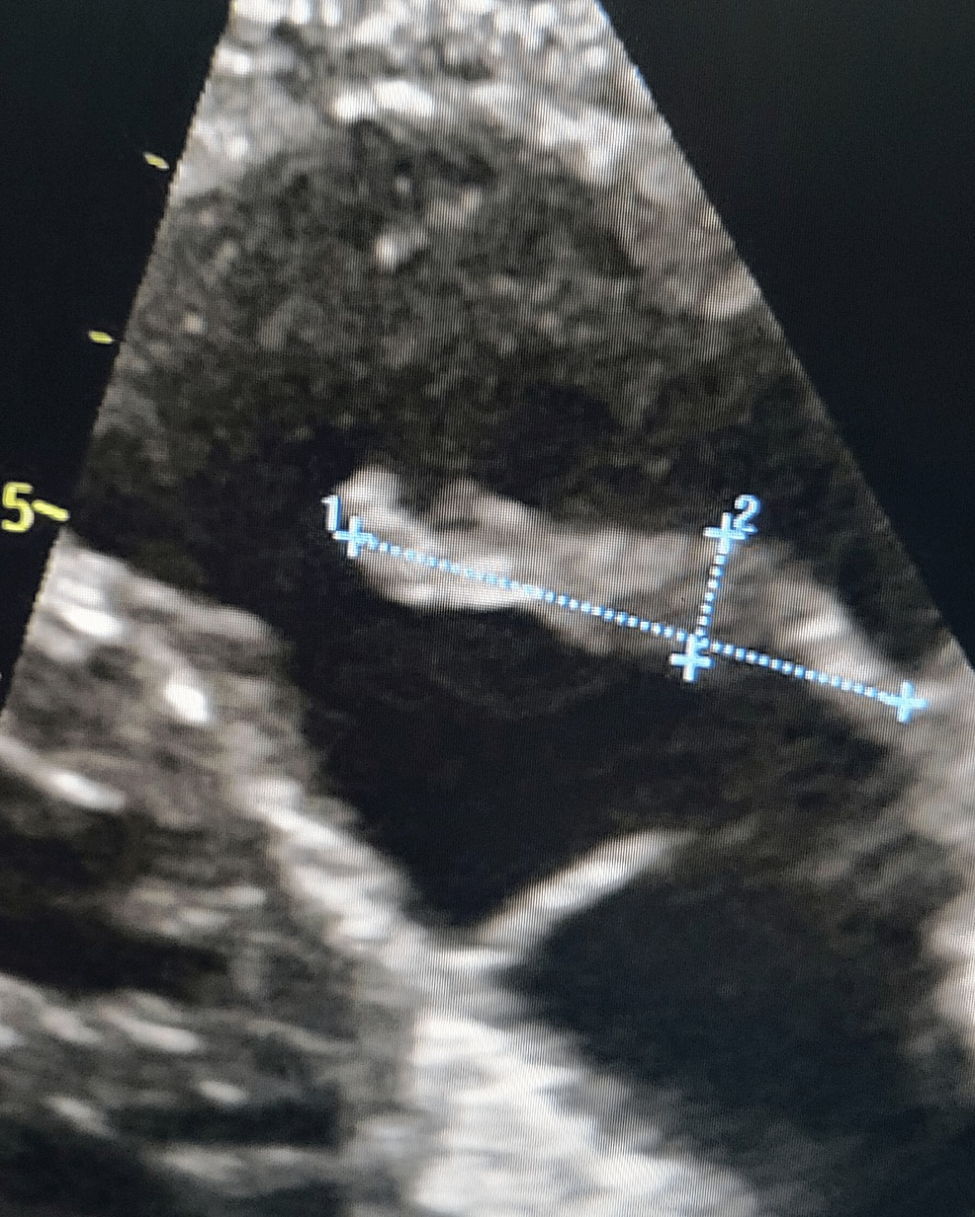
TTE showing the pulmonary valve vegetation measuring 3.2x1.1 cm TTE: transthoracic echocardiography
